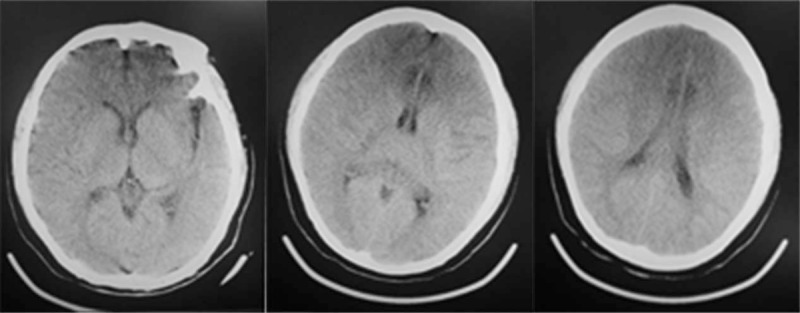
Cranial CT showing diffuse low density image, suggesting cerebral edema. CT = computed tomography.
